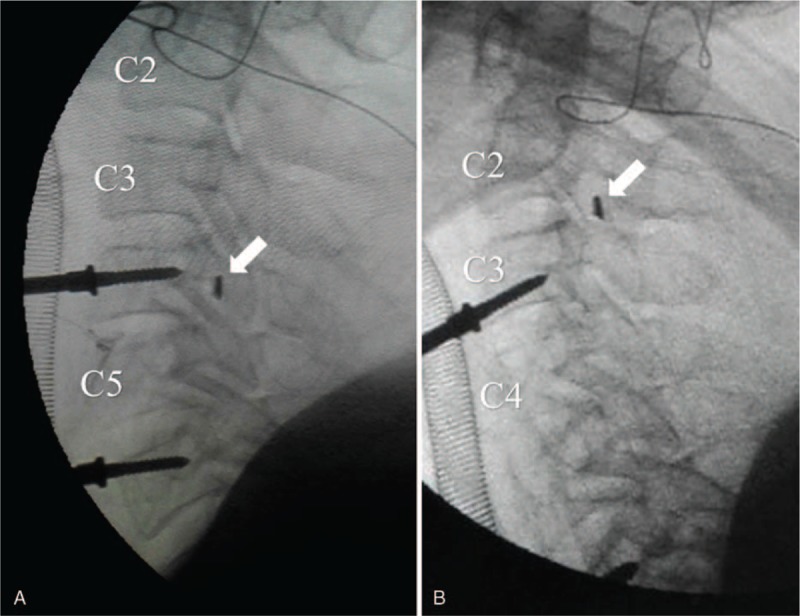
Intraoperative x-ray revealed the broken metal tip (arrow) was located behind C4 vertebral body (A). After C4 vertebral body was removed in the remedy C4 corpectomy, however, the metal fragment was found to migrate to C2/3 canal (B).
